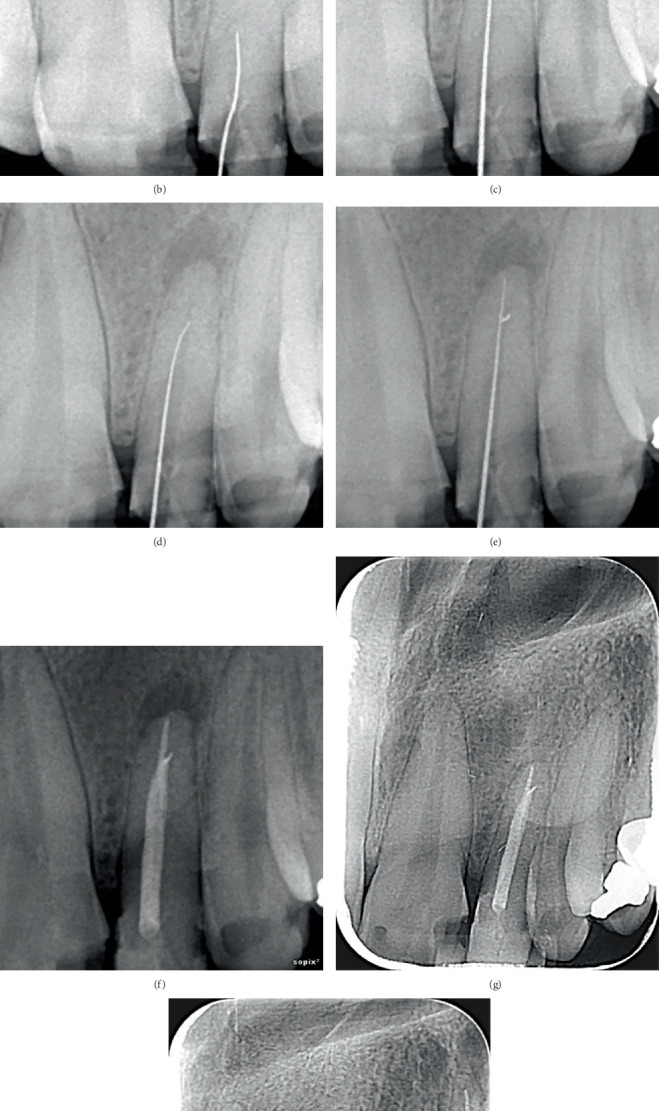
(a) Pretreatment CBCT imaging of the tooth. (b) Minor transportation was observed after initial attempts at root canal negotiation. (c, d) Further attempts to reach the main root pathway. (e) Successful negotiation of the root canal and a remaining separated file. (f) Final obturation of the canal. (g) Periapical radiograph at the 6-month follow-up visit. (h) Periapical radiograph at the 13-month follow-up visit.
